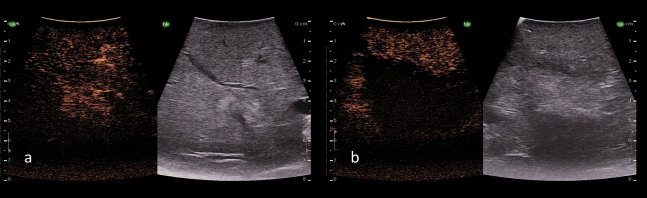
Intraoperative ultrasound of a tumor using a microbubble contrast agent, a) Before thermal ablation the tumor enhances on contrast ultrasound demonstrating viable cells with blood flow, b) After thermal ablation blood flow can no longer be appreciated in the tumor and it appears dark on contrast ultrasound.
